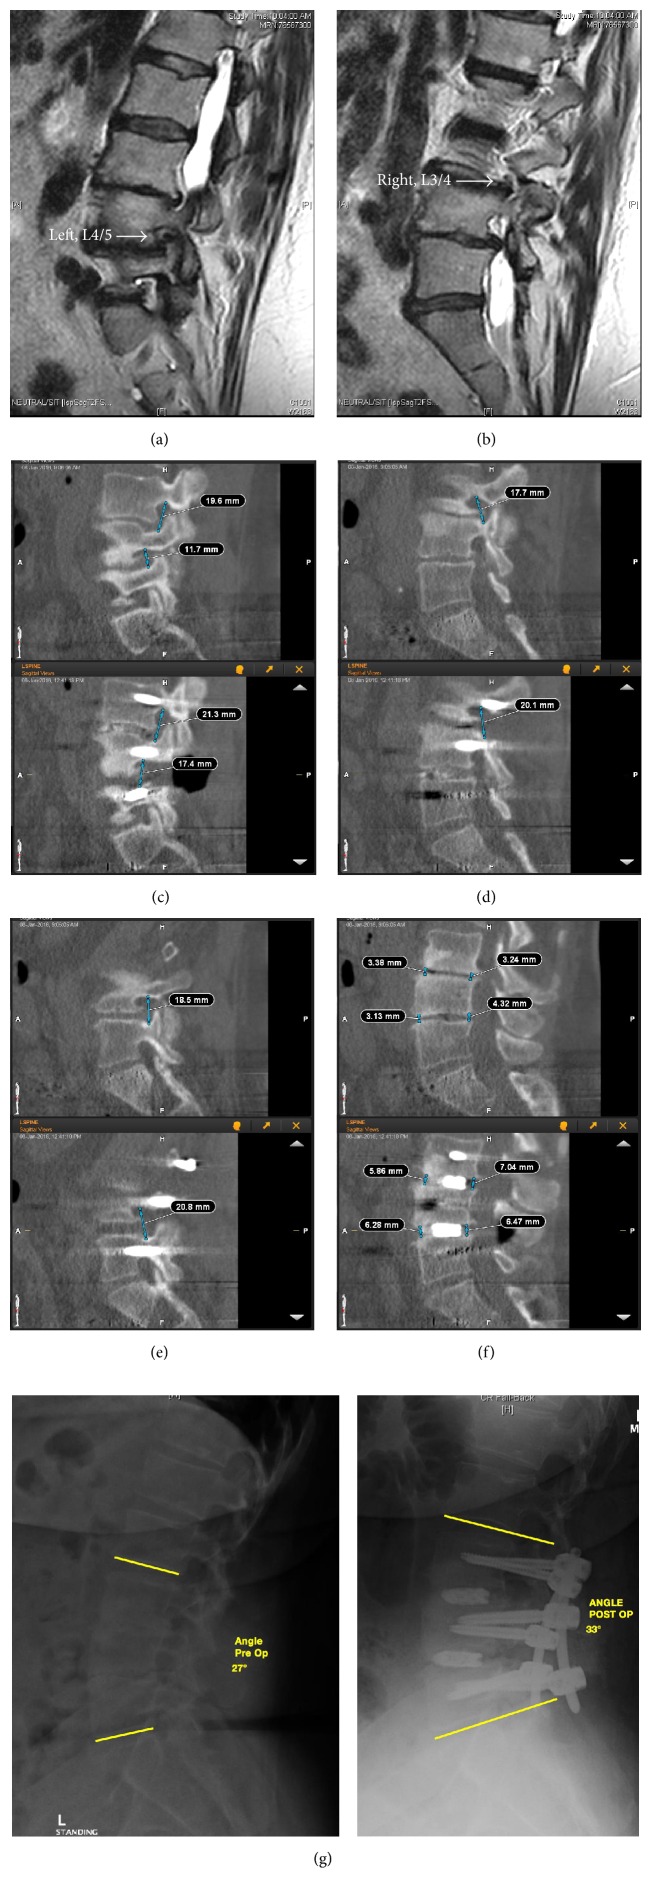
A 63-year-old female with two-level stenosis and instability. Pre-op MRI showed foraminal stenosis of left side in L4/5 (a) and right side in L3/4 (b). From navigation screen, foraminal heights were measured preoperatively and postoperatively. L3/4 and L4/5 of left side increased from 19.6 mm and 11.7 mm to 21.3 mm and 17.4 mm, respectively (c). L3/4 of right side increased from 17.7 mm to 20.1 mm (d). L4/5 of right side increased from 18.5 mm to 20.8 mm (e). The disc height of L3/4 and L4/5 increased postoperatively (f). The local lordosis improved postoperatively (g).
